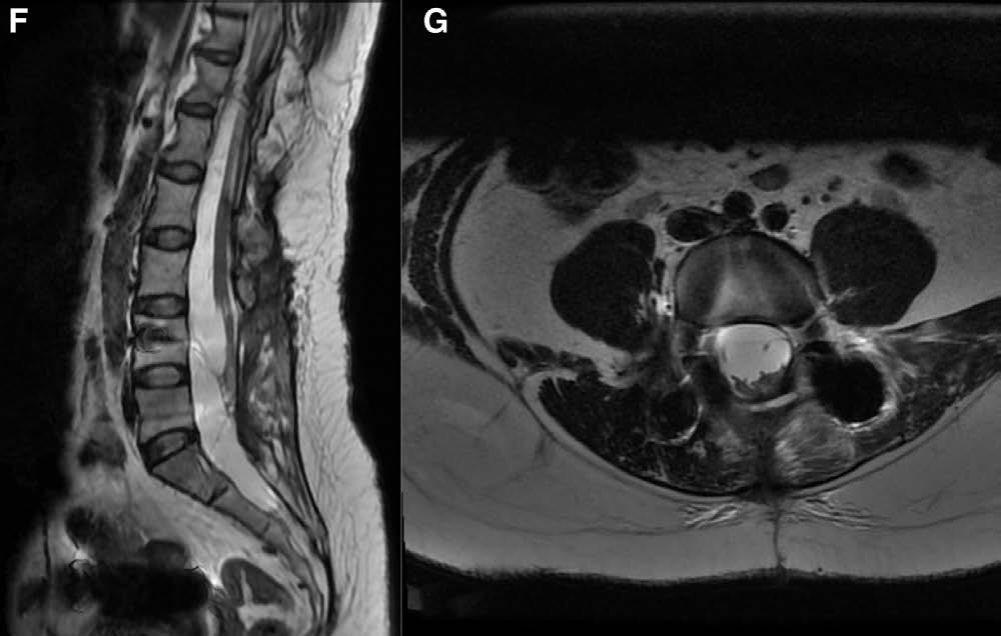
(F and G) Images taken 2 y after the surgery. The lumbar spine plain MRI scan in sagittal section (F) and cross-section (G). MRI = magnetic resonance imaging.
